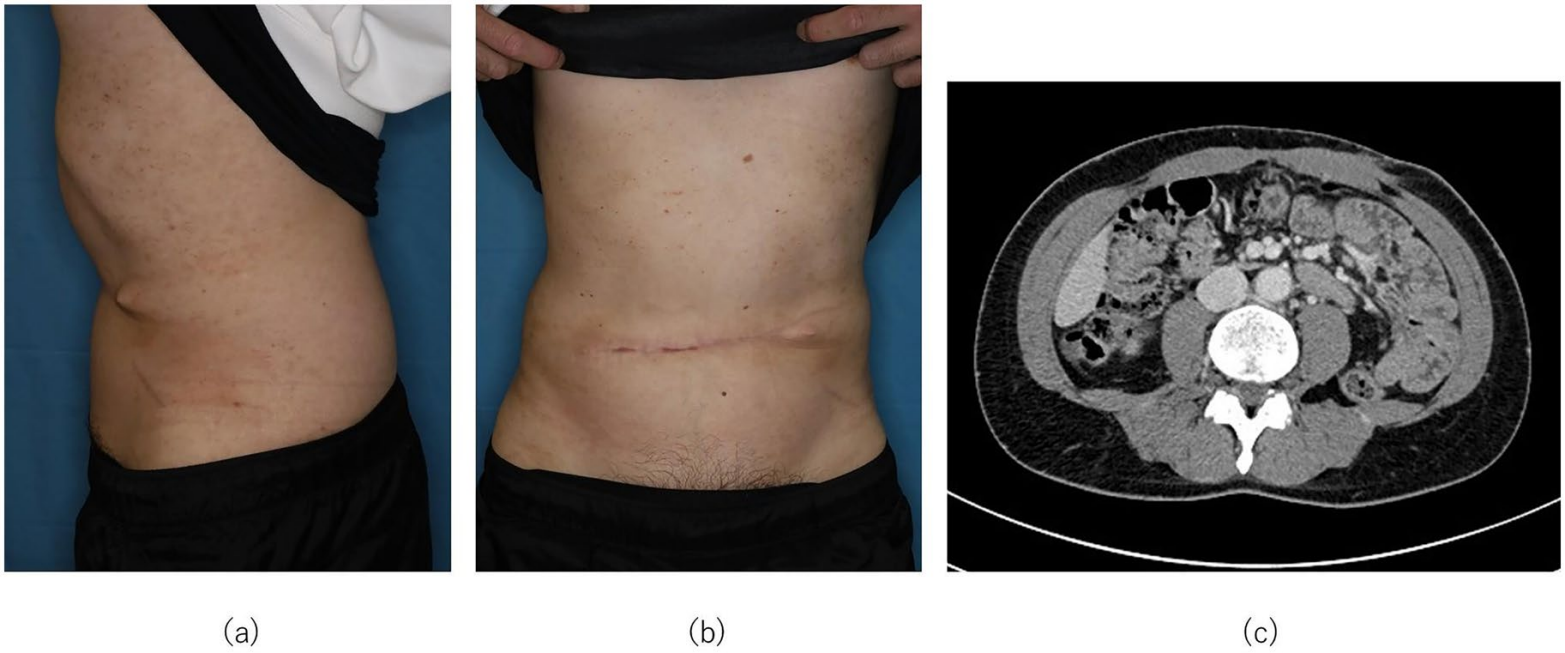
Six months postoperatively. (a) Lateral view showing a flat abdominal contour without evidence of hernia. (b) Frontal postoperative photograph demonstrating no bulging at the umbilical site. (c) No evidence of hernia was observed on follow-up CT imaging.
